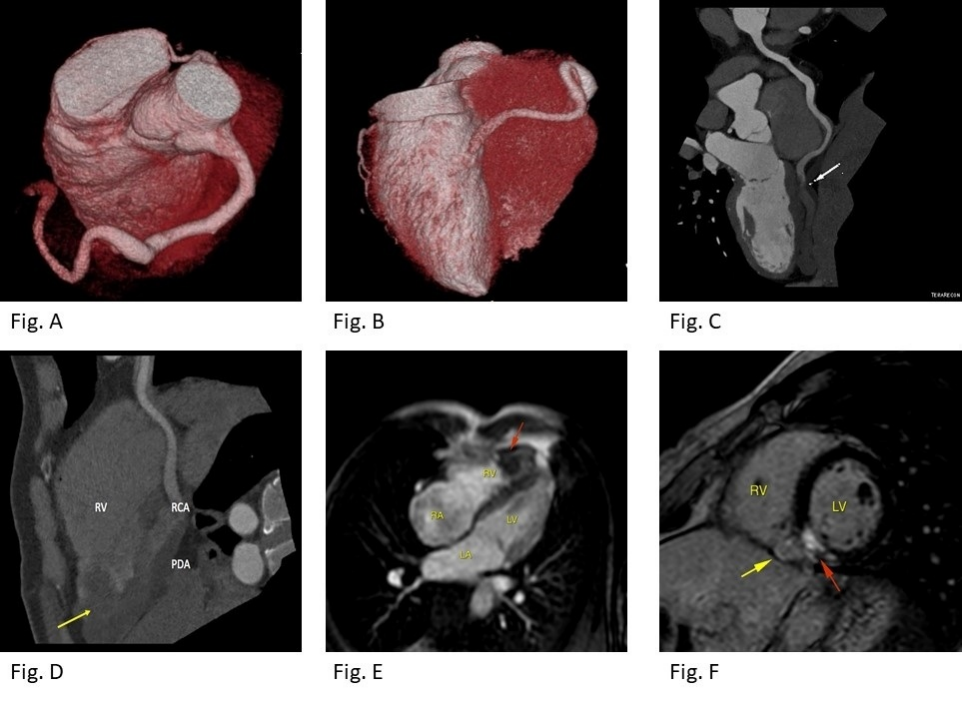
Cardiac computed tomography (CCT) A and B: CCT three-dimensional reconstruction (volume rendering technique) demonstrates an aneurysm in right coronary artery (RCA) with total oclusion in the distal segment. C and D: Curved multiplanar reformation (MPR) shows thrombus in distal RCA and posterior descending artery (PDA) (white arrow) with extension to the right ventricle (RV) (yellow arrow). E. Long-axis of first-pass myocardial perfusion on cardiac magnetic resonance imaging (MRI) demonstrates a large thrombus image inside the RV. F. Left ventricle (LV) late gadolinium enhancement short-axis on cardiac MRI. Note delayed enhancement in the LV inferoseptal segment of basal region (red arrow) and right ventricle involvement (yellow arrow) compatible with myocardial infarction. RA: right atrium; LA: left atrium.
